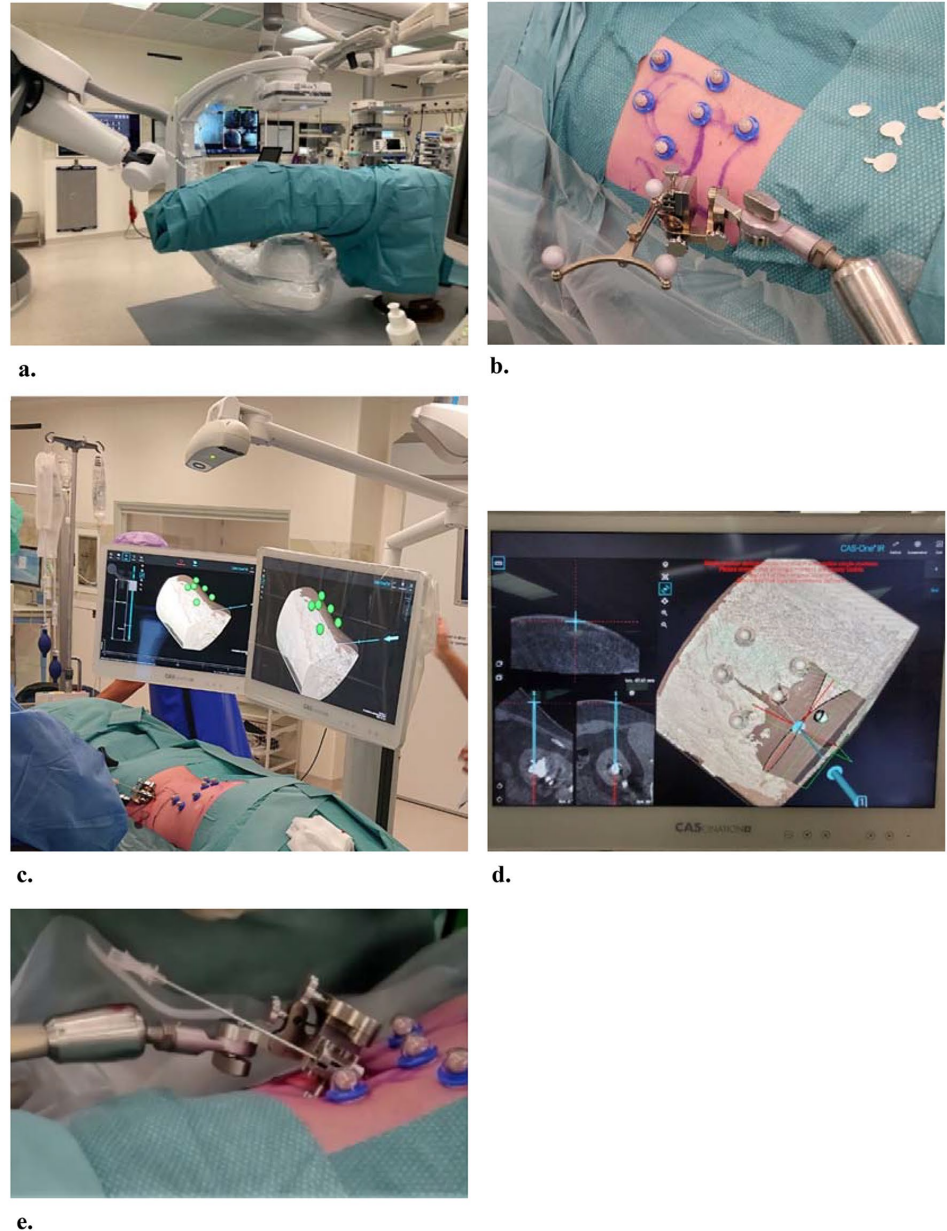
Stereotactic optical navigation for needle guidance in the percutaneous nephrolithotomy procedure. a Overview of the operating room with the patient prepared for cone-beam CT imaging. b Photograph of the fiducial markers on the skin, positioned around the expected needle entry point, and on the aiming device, which is positioned in the needle guide. These markers are used for image-to-patient registration. c Overview of the set-up for optical navigation with the CAS-One IR system. The camera detects the markers on the skin and correlates this with the cone-beam CT scan. The markers are shown as green spheres in the scan. d Using the navigation software, the needle tract is planned by selecting an entry point on skin level and a target point in the selected calyx. e After aligning the aiming device with the planned trajectory, the needle is inserted manually through the needle guide
